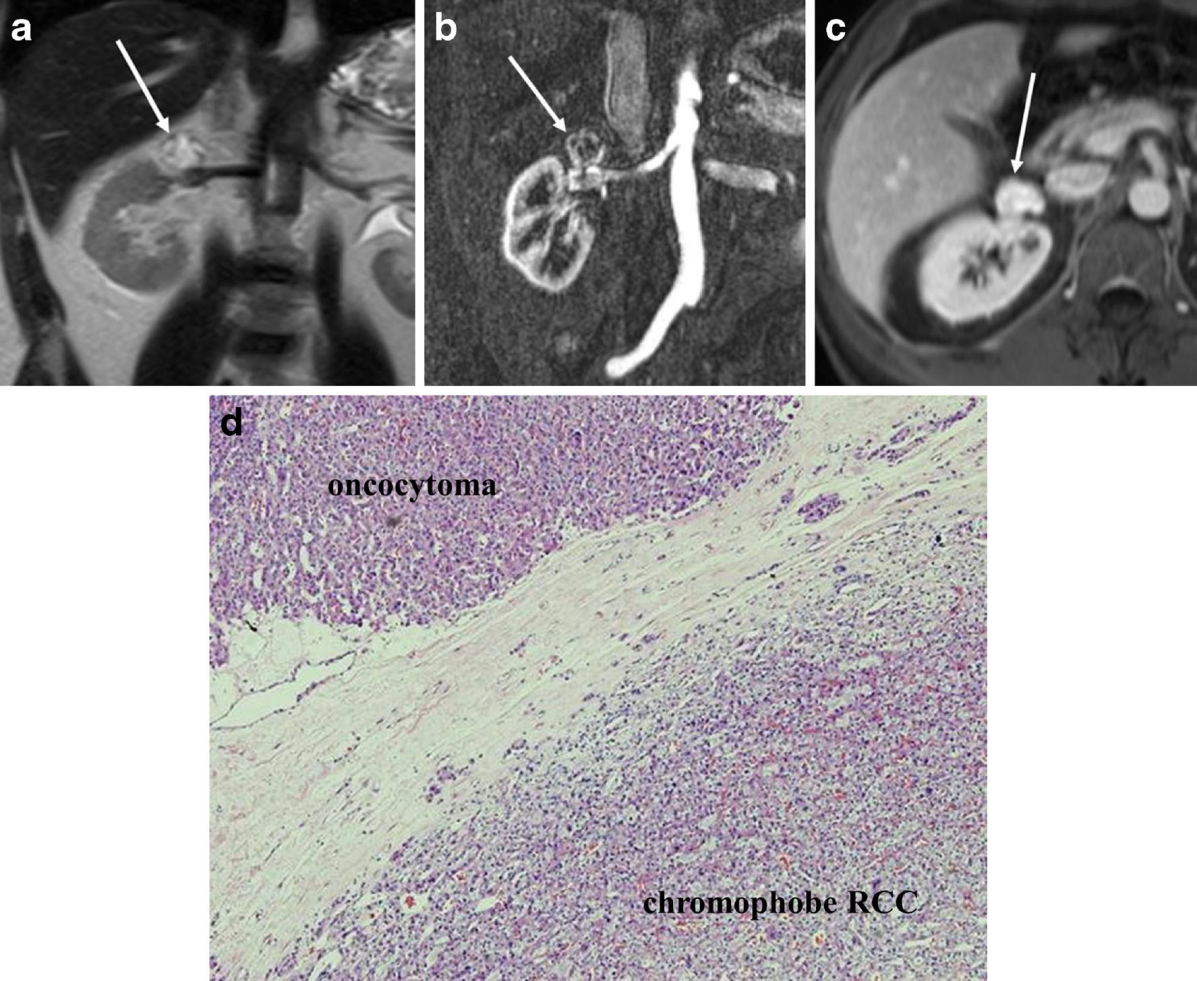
A 54-year-old man with a hybrid tumour (oncocytoma containing chromophobe RCC component) in the right kidney. a The coronal T2-weighted single shot FSE MR image shows a right upper renal pole mass with mixed high and intermediate signal intensity (*arrow*). b The corticomedullary phase of the coronal T1-weighted GRE image shows heterogeneous enhancement of the mass. c The axial post-contrast T1-weighted GRE image with fat saturation shows persistent and homogenous enhancement of the mass (*arrow*). This oncocytoma also demonstrates a lobular morphology. d (Low magnification) Hybrid tumour: *upper left* oncocytoma; *lower right* eosinophilic variant of chromophobe renal cell carcinoma (chromophobe RCC)
